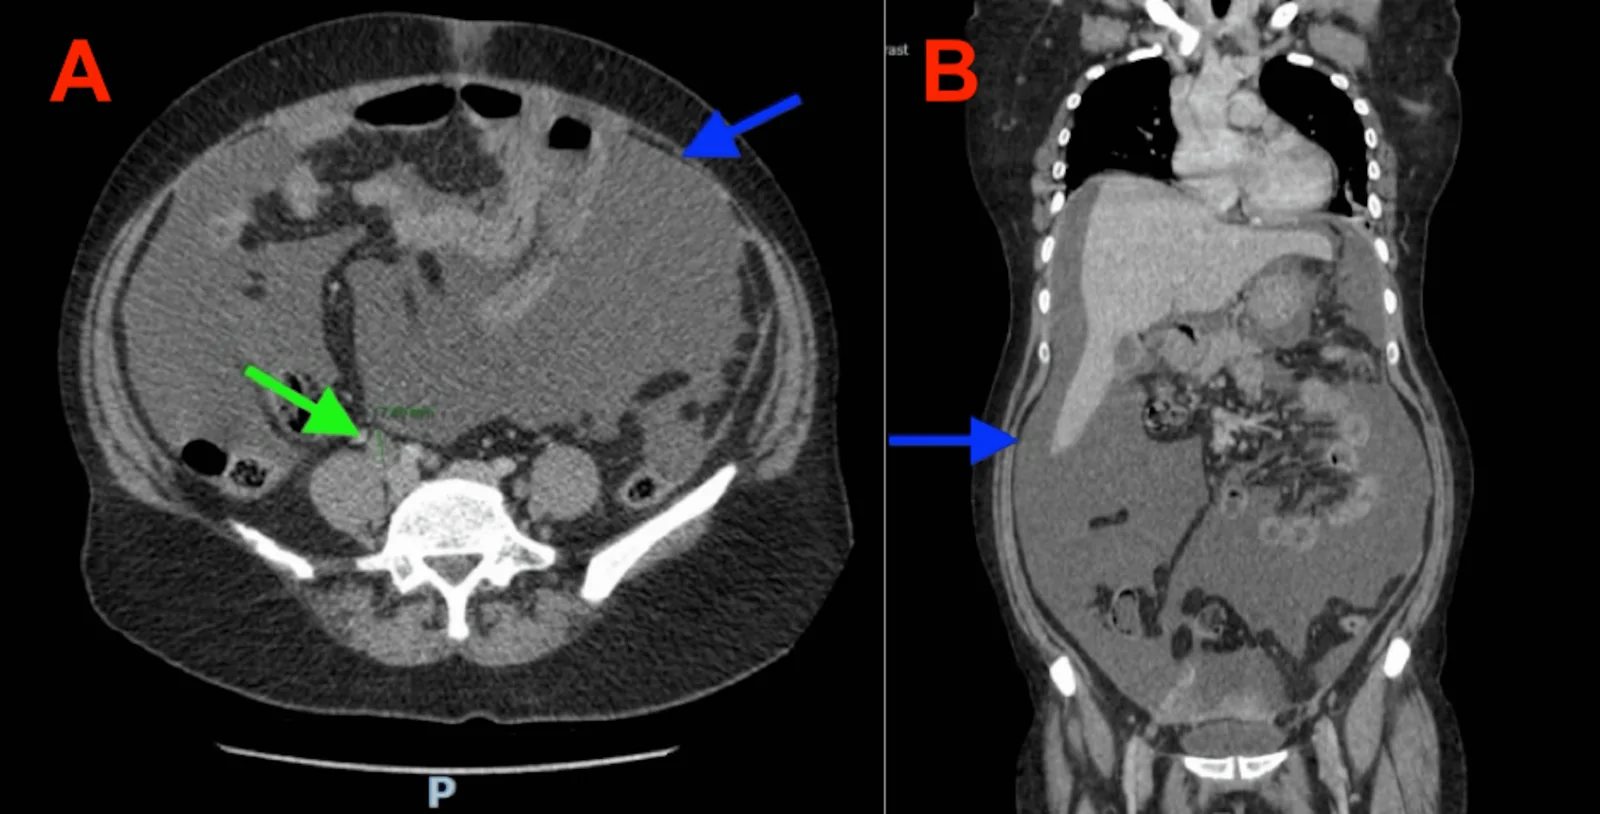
CT Chest, abdomen and pelvis with contrast (08/2022) - transverse (A) and coronal (B) sections, showing significant ascites with omental caking (blue arrows), and enlarged retroperitoneal lymph nodes (green arrow)
